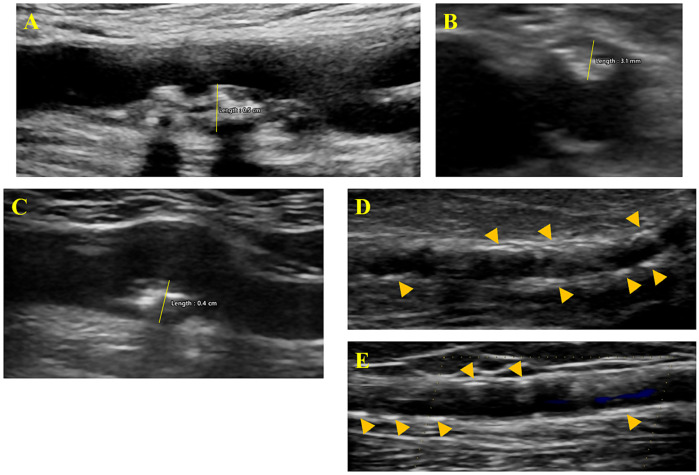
Vascular ultrasound assessment—measurement of femoral plaque thickness in longitudinal (A) and axial (B) view; measurement of carotid plaque thickness in longitudinal view (C); identification of distinct radial artery calcifications—arrow heads (D,E).
